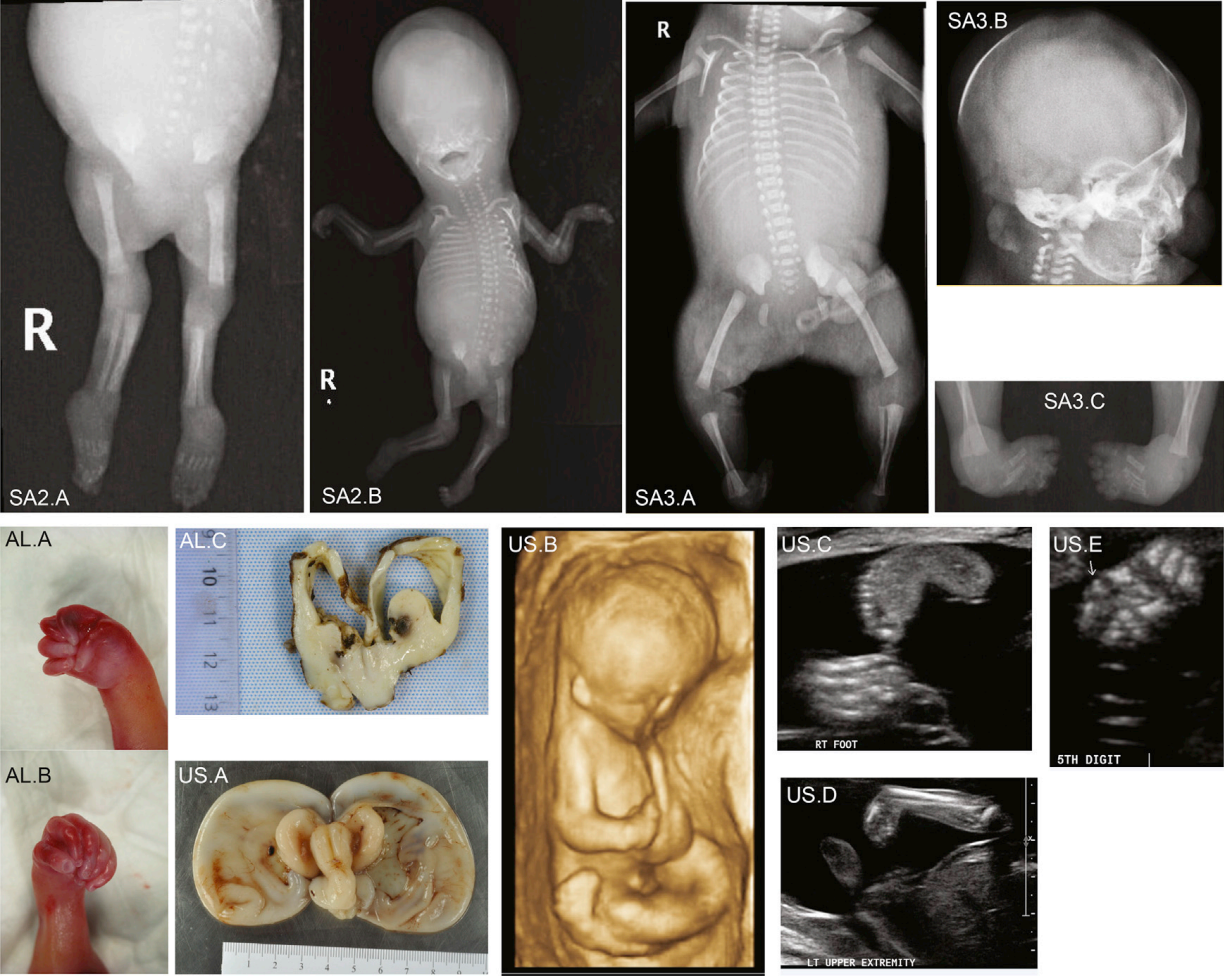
Ultrasound, X-Rays, and Autopsy Images of the SA2.II.1, SA3.II.1, AL.II.1, and US.II.3 Fetuses X-ray images showing arthrogryposis of SA2.II.1 fetus (SA2.A and SA2.B). X-ray images showing SA3.II.1 skeleton (SA3.A), head (SA3.B), and club feet (SA3.C). Autopsy pictures from the AL.II.1 fetus showing right (AL.A) and left (AL.B) adductus thumbs of the fetus, and dilatation of cerebral ventricles with agenesis of corpus callosum (AL.C). Autopsy image of the brain from US.II.3 fetus showing hydrocephalic brain with diaphanous pallium (US.A). The colliculi appear as single elongated ridges separated by a midline futter and the midline appears angulated on the brainstem, which is small as is the cerebellum. Antenatal ultrasound scan showed general arthrogryposis (US.B), one hyperflexed wrist (US.C), club feet (US.D), and bilateral clinodactyly of one hand (US.E).
